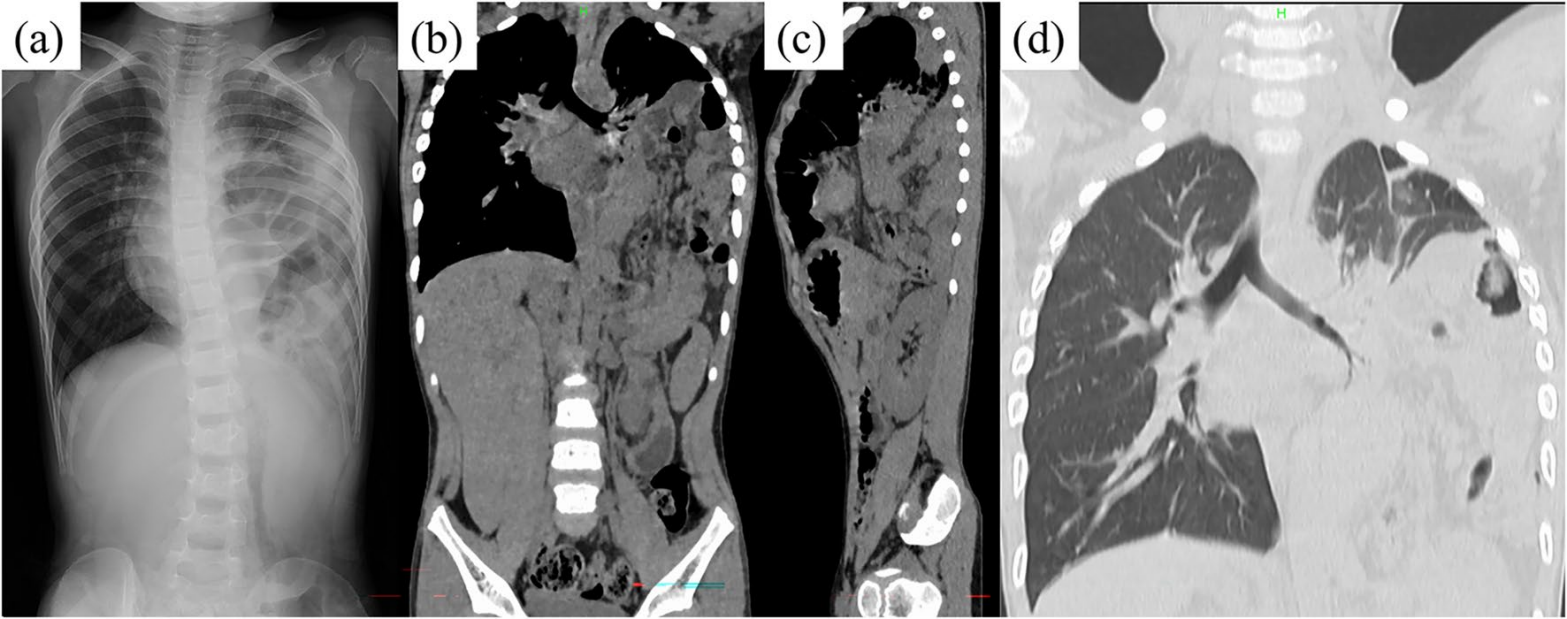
Findings of preoperative imaging. a Thoracoabdominal X-ray showed a herniated intestine in the left thoracic cavity and no intestinal gas in the abdomen. The coronal section (b) and sagittal section (c) and coronal section of pulmonary window setting (d) of plain thoracoabdominal CT showed herniation of the small intestine and colon into the left thoracic cavity. The spleen and stomach were not herniated. Atelectasis of left lung was mild
